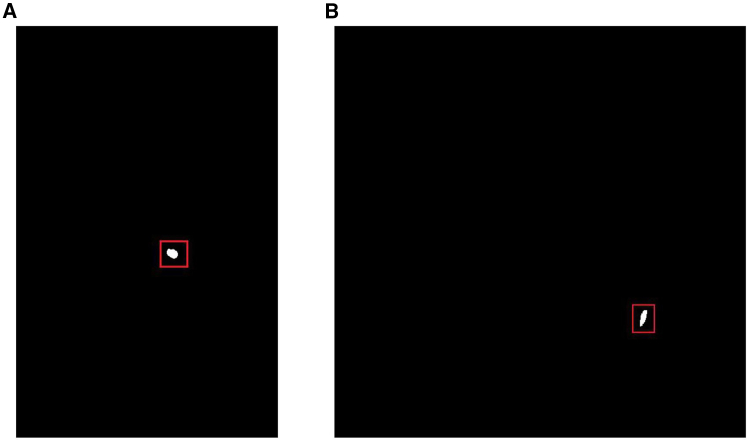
Example of small lesions CT (A) Segmentation of a small. (B) Segmentation of an elongated.
